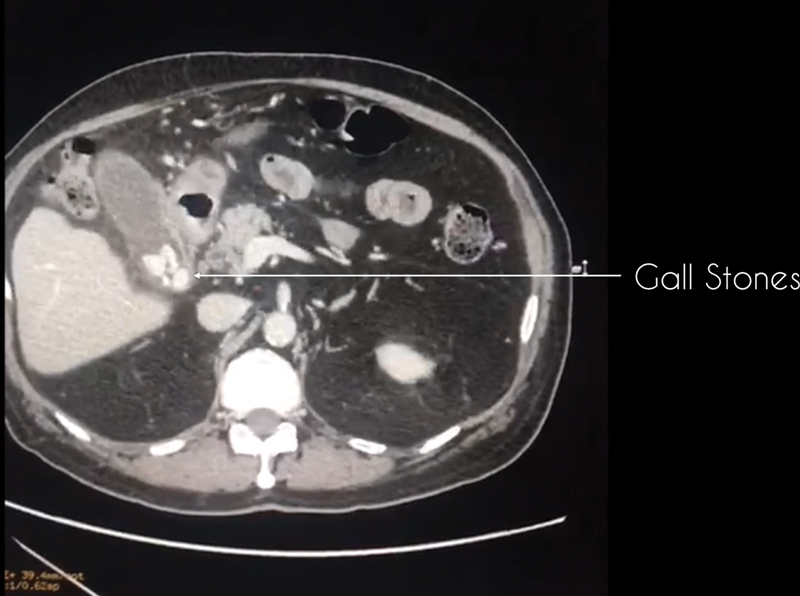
CT image showing gall stones and surround peri gall bladder fat stranding. CT, computed tomography.
